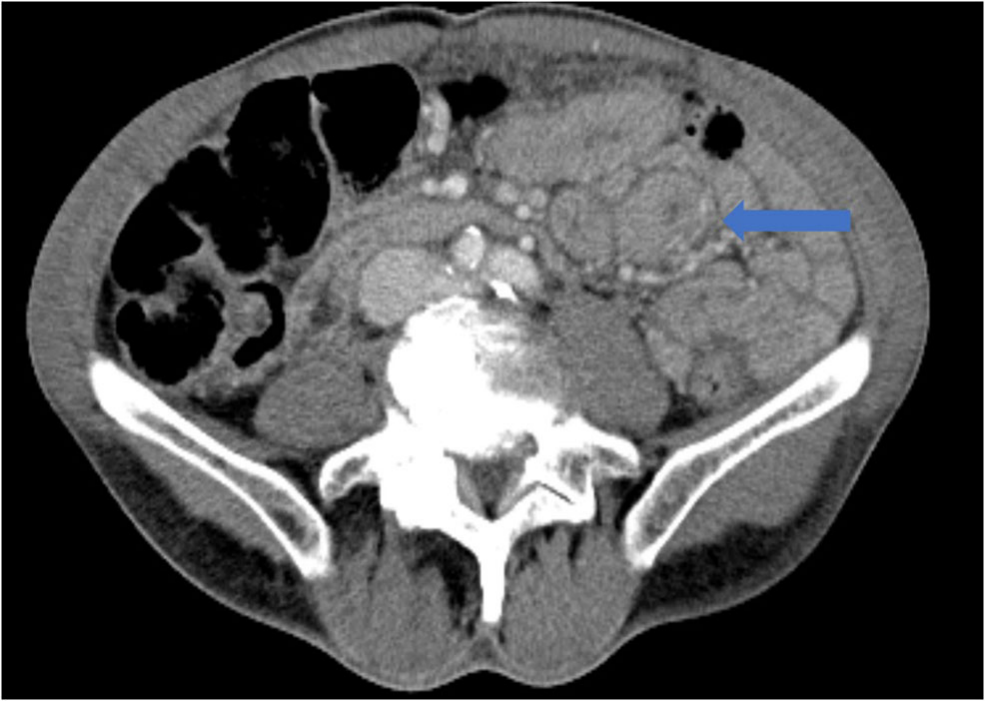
Axial post-contrast CT scan demonstrates diffuse bowel wall thickening of the distal duodenum and jejunum (blue arrow), indicative of gastroenteritis.
